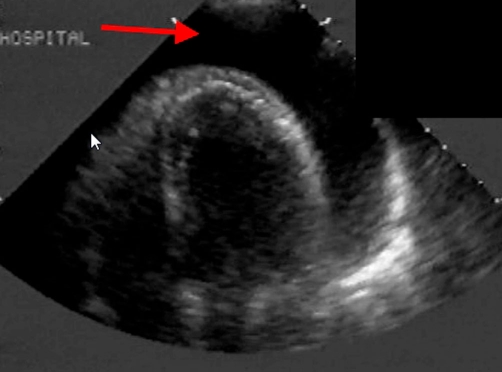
Echocardiographic image. Confirmation of a large pericardial effusion shown on this echo image by the red arrow.
